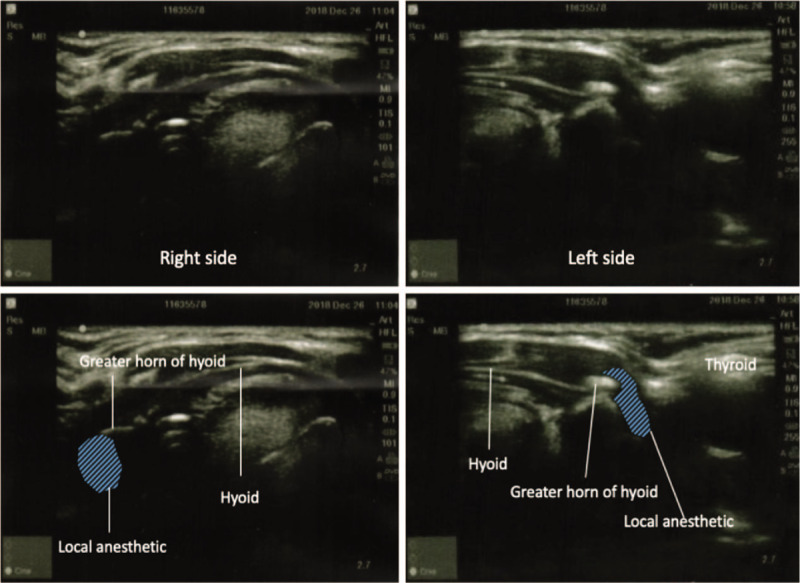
Ultrasound-guided superior laryngeal nerve block performed through a transverse approach. A 1.5-ml injection of 2% lidocaine was performed around the greater horn of the hyoid bone, which was identified as the sonographic landmark.
